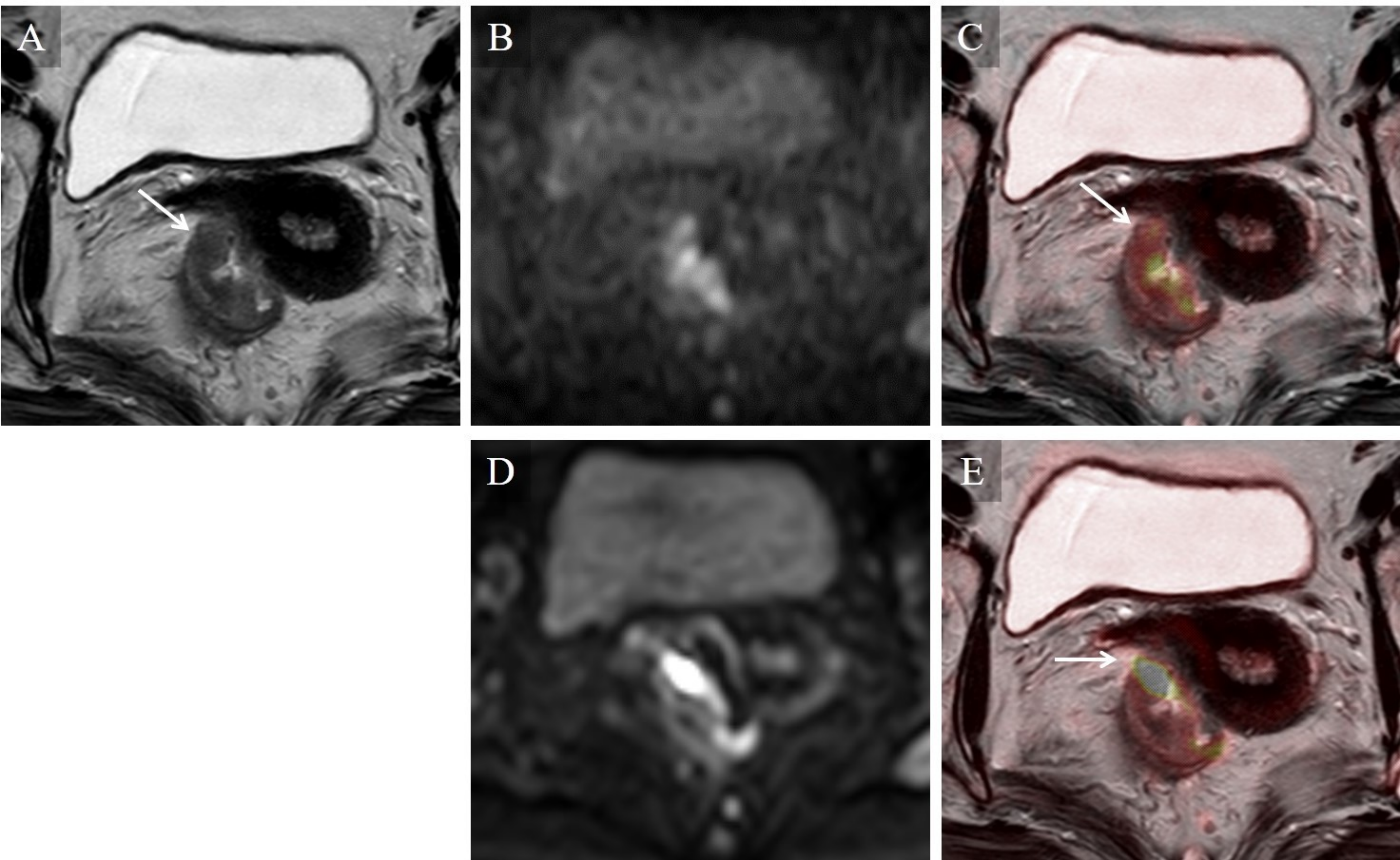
Stage T3 rectal carcinoma in a 69-year-old woman. On the T2WI image (A) alone, intermediate intensity of tumor was shown beyond the muscularis propria (arrow). Higher image noise was seen on the TSE-DWI image (B) than on the EPI-DWI image (D), but this did not degrade the fusion image of TSE-DWI (C). On the fusion image of TSE-DWI (C), a colored area of diffusion restriction corresponded to the low intensity area on the T2WI-presenting tumor (arrow). On the fusion image of EPI-DWI (E), a colored area of diffusion restriction was depicted beyond the low intensity area on T2WI (arrow) due to distortion artifacts, but did not degrade the T-staging.
